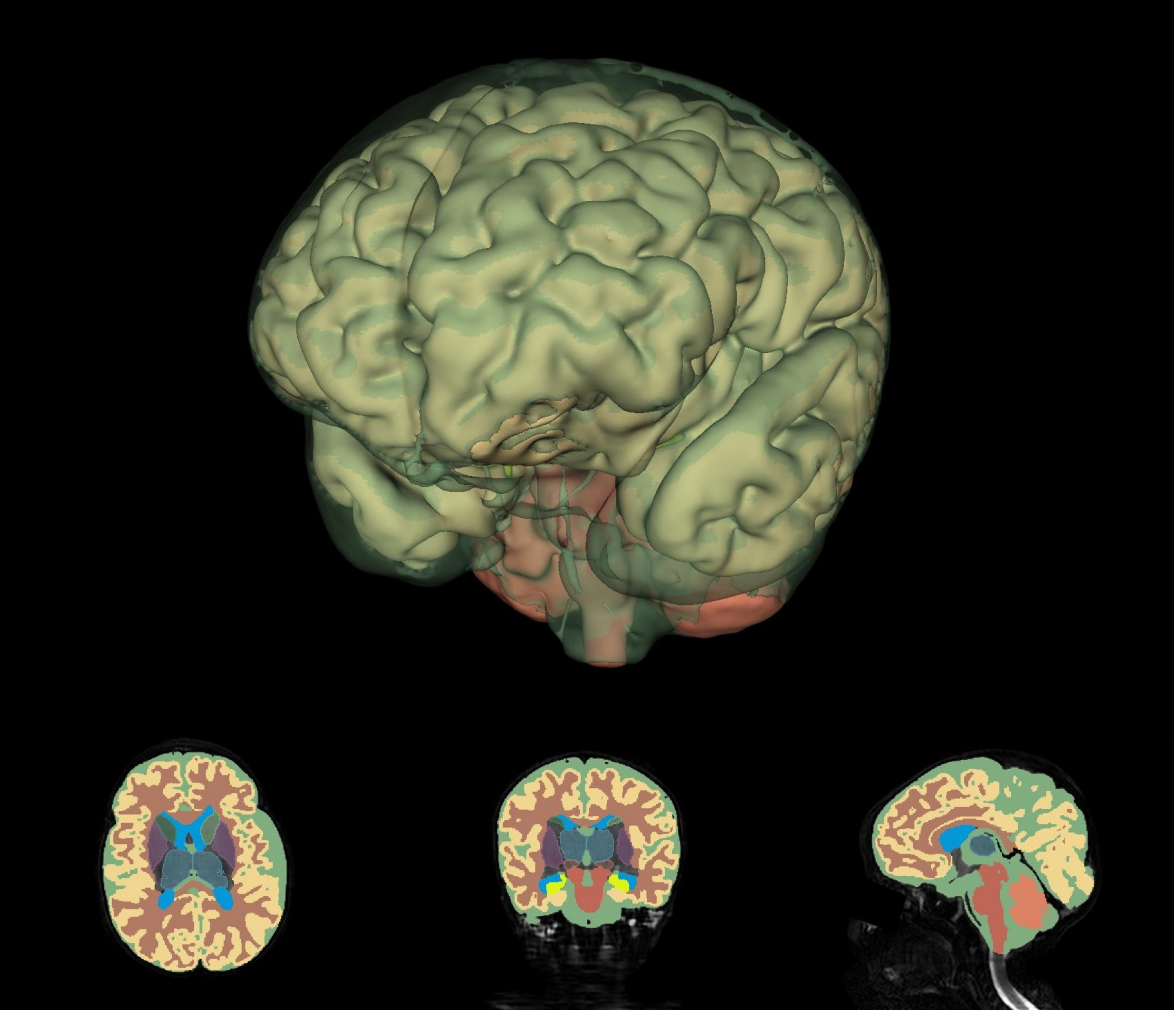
Example 3D reconstruction of an acquired brain MRI image and regional segmentations. A 3D reconstruction (top) of the MRI‐derived regional brain segmentations with CSF (semitransparent), cortex, brainstem, and cerebellum visible. Axial (bottom left), coronal (bottom middle), and sagittal (bottom right) views showing MRI‐derived regional brain segmentations, including CSF (green), cortical gray matter (yellow), white matter (red), ventricles (blue), and deep gray matter structures (caudate nuclei, thalami, subthalamic nuclei, and lentiform nuclei). 3D indicates 3‐dimensional; CSF, cerebrospinal fluid; and MRI, magnetic resonance imaging.
